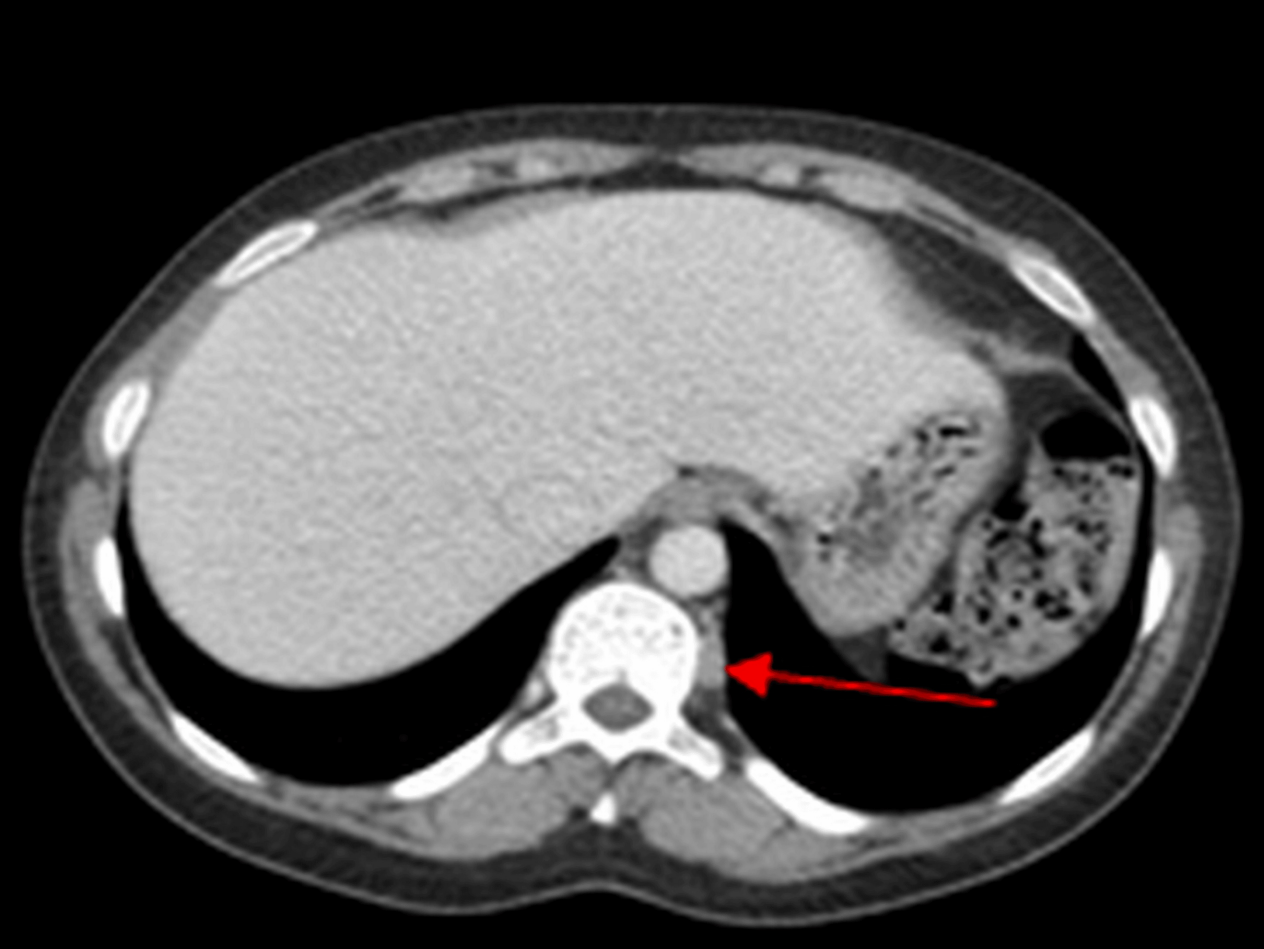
Computed tomography (CT) image from 2013 demonstrating the paravertebral lesion (arrow).
